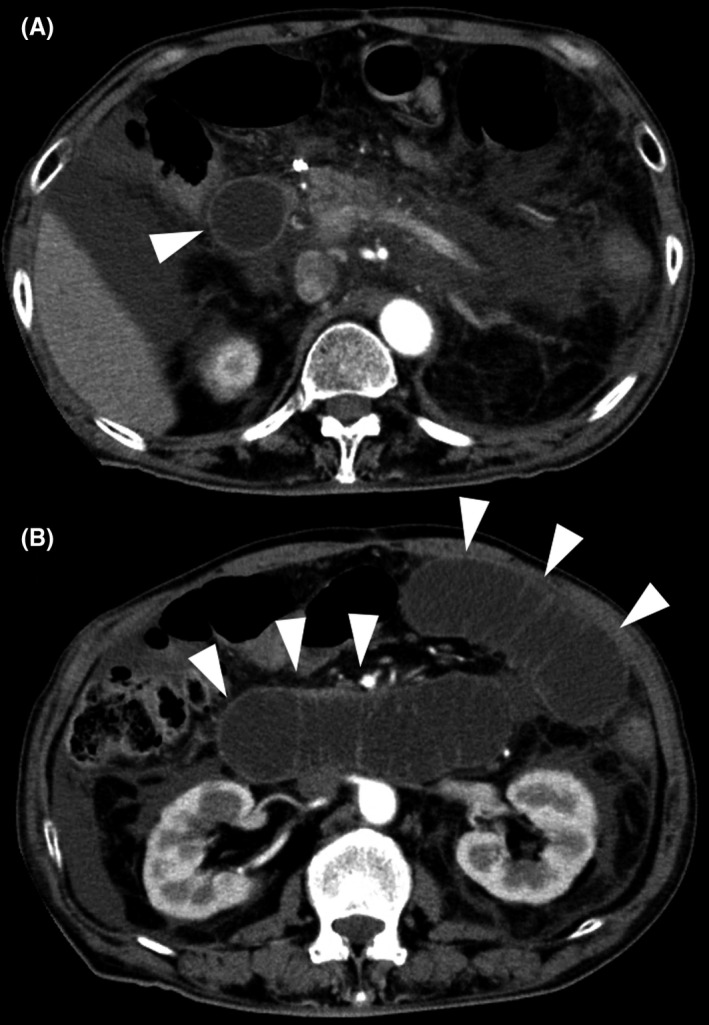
(A and B) Contrast‐enhanced computed tomography revealed swelling in the pancreas and a dilated afferent loop (arrow heads)
